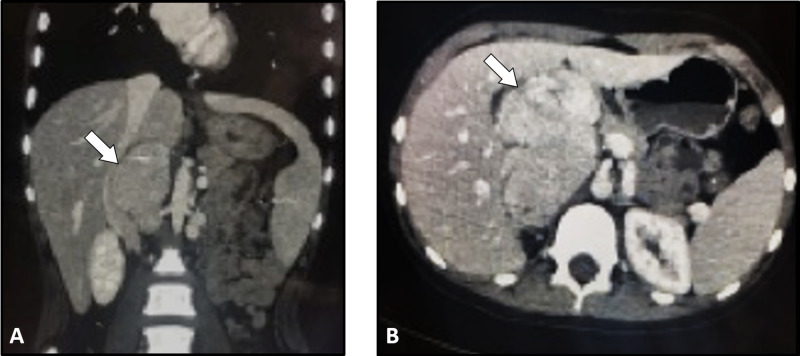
Abdominal CT images Coronal (A) and axial (B) CT images showing a mess lesion located superior to the right kidney. No evidence of thrombosis or lymph node enlargement is noted.
